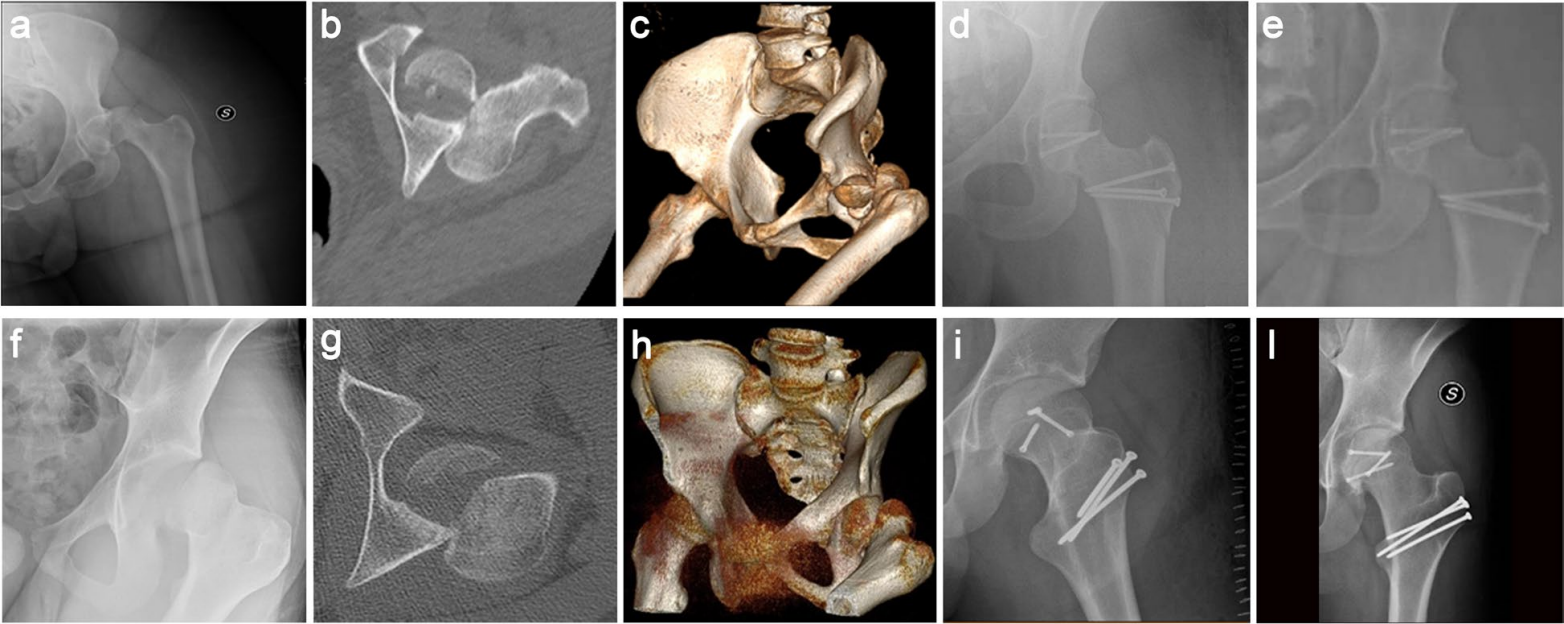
A 33-year-old woman (a-e) and a 17-year old man (f-i) with left femoral head Pipkin type II fracture treated by ORIF through Gibson approach and surgical dislocation with Ganz trochanteric flip osteotomy. The preoperative antero-posterior (AP) hip radiograph, axial CT and CT 3-D reconstruction after injury (a-c, f-h) and post-operative and final follow-up radiographs (d, e, i, l)
